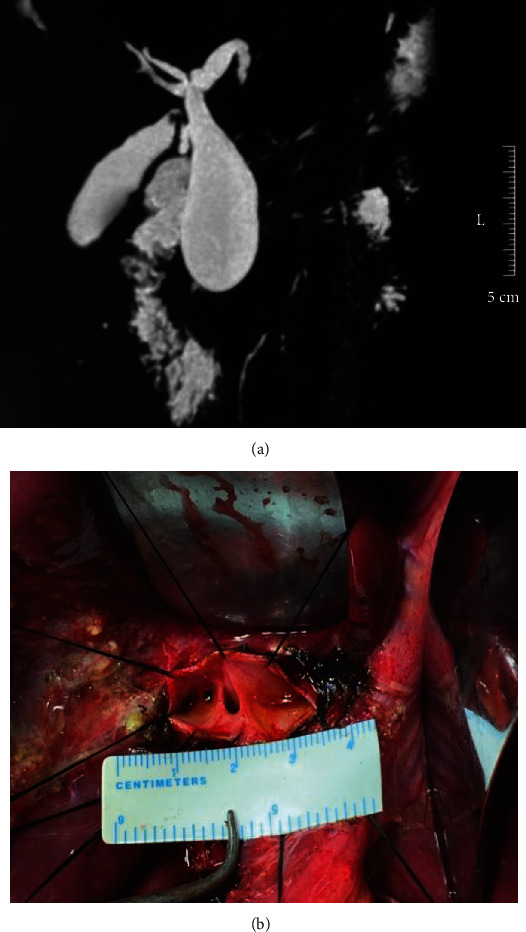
(a) Ultrasonographic image of type Ia CDBD. (b) Completed appearance of a hilar ductoplasty in type Ia CDBD.
